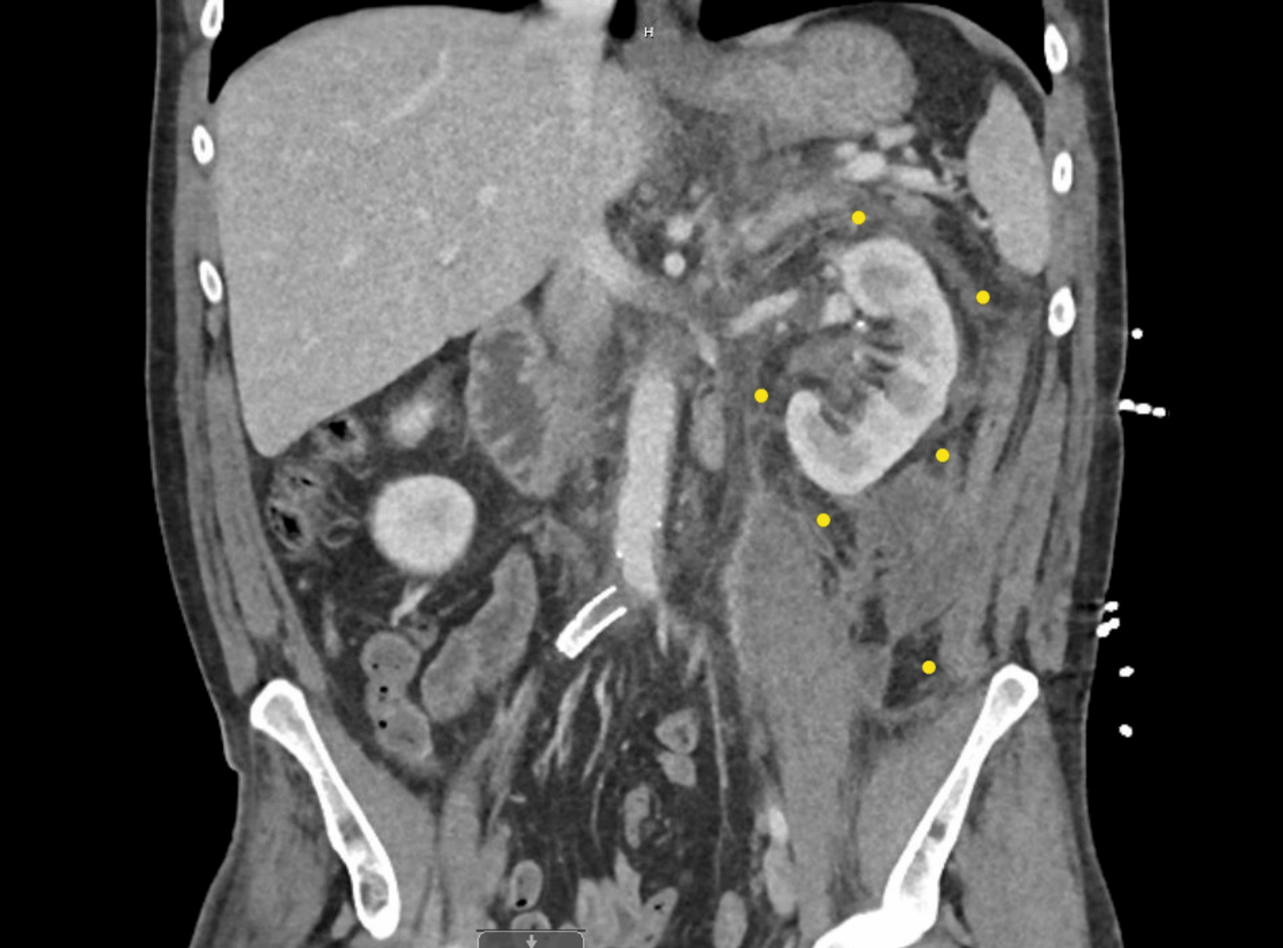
CT Abdomen Redemonstrating the Perinephric Fluid Collection in the Left Perinephric Space CT scan of the abdomen in coronal plane showing extensive fluid collection in the left perinephric space highlighted by yellow markings.
